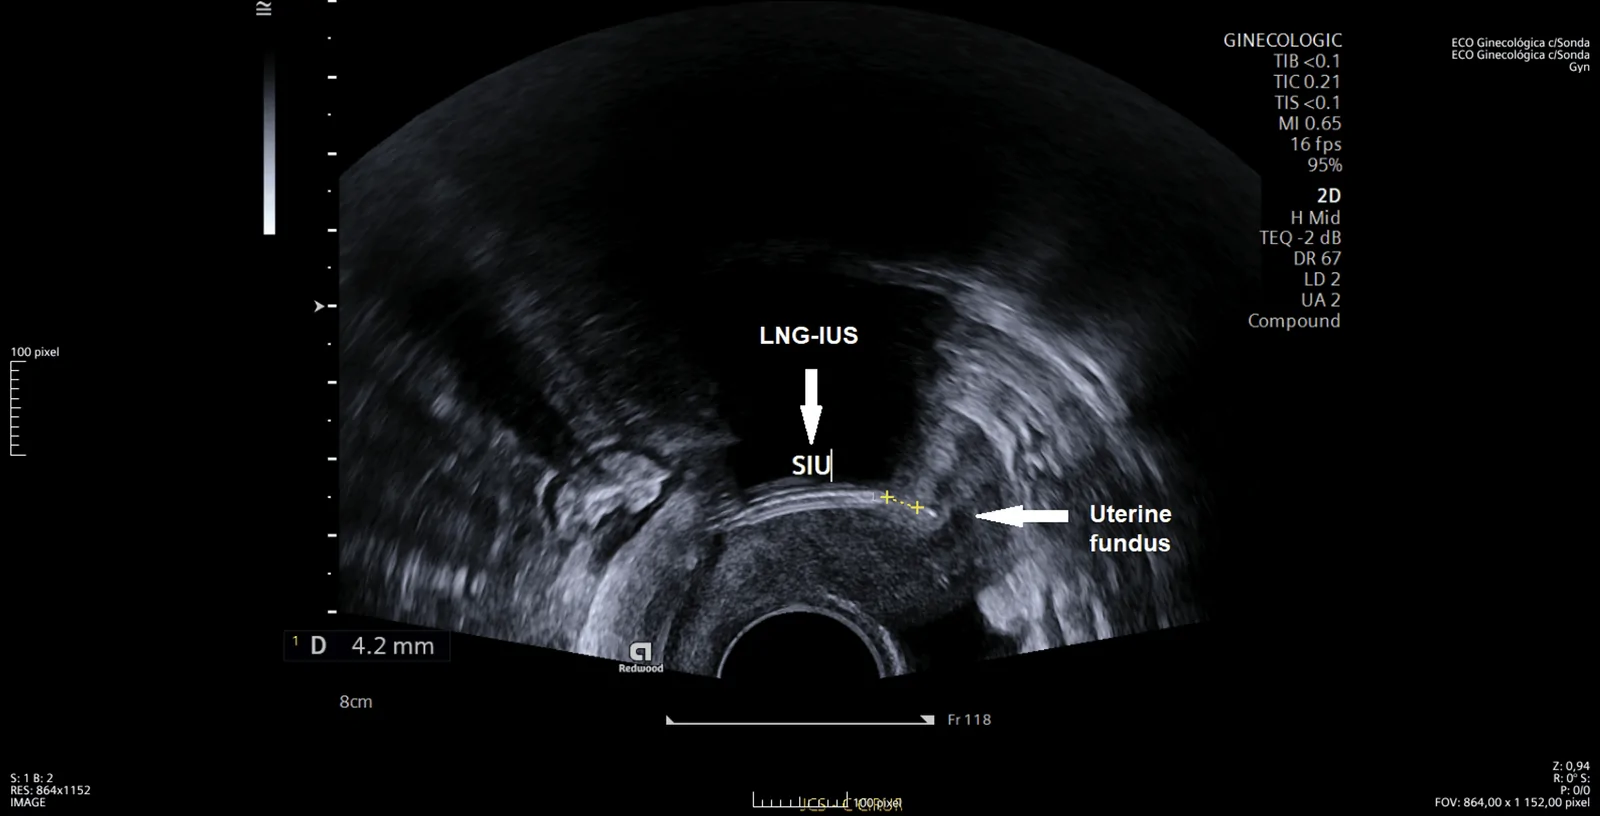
Follow-up TVUS (sagittal view) demonstrating correct fundal positioning of the LNG-IUS within a retroflexed uterus following the successful repeat insertion. The white arrows indicate the LNG-IUS (left) and the uterine fundus (right). TVUS: Transvaginal ultrasound; LNG-IUS: Levonorgestrel-releasing intrauterine system
